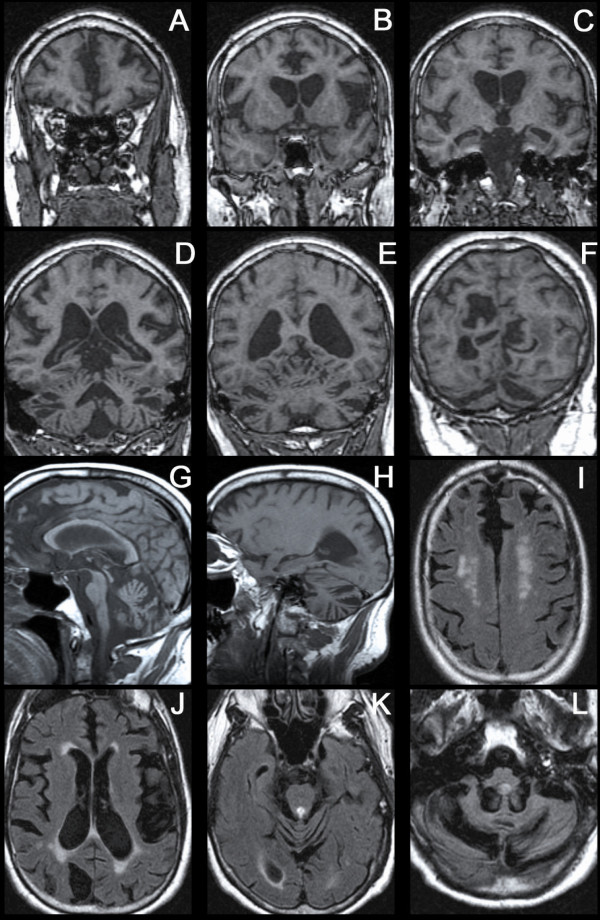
MRI at age 61 demonstrating severe atrophy of the cerebral hemispheres, cerebellum, and brainstem. A-F: 3-D SPGR coronal MRI; G, H: T1 sagittal MRI; I-L: FLAIR axial MRI, showing white matter signal hyperintensities.
